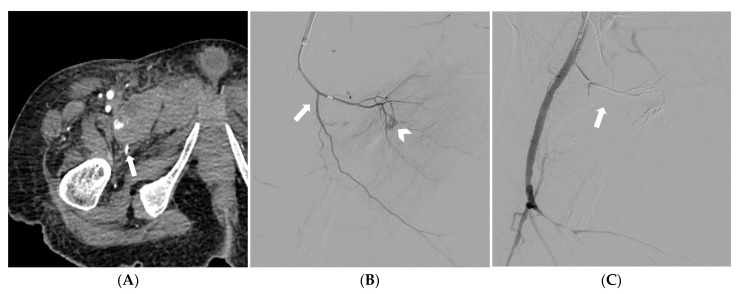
CT angiography depicting a spontaneous pectineus muscle hematoma with active bleeding (arrow) (A). Selective catheterization of the right profunda femoris artery and superselective catheterization of the medial circumflex femoral artery (arrow); at digital subtraction angiography active bleeding is noted (arrowhead) (B). Digital subtraction angiography demonstrating successful embolization with a gelatin sponge (arrow); preserved patency of the profunda femoris artery and its other branches is also noted (C).
